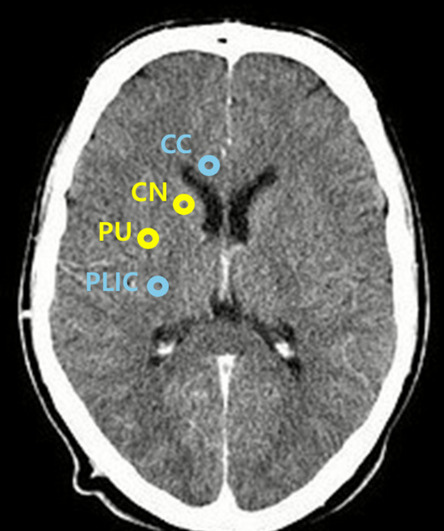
The GWR measurement at the basal ganglia level using brain CT. The calculation methods are as follows: BG-GWR = (CN + PU)/(CC + PLIC); simplified BG-GWR = PU/PLIC. Abbreviations: CT, computed tomography; GWR, gray-to-white matter ratio; BG-GWR, GWR measured at the basal ganglia level; CC, corpus callosum; CN, caudate nucleus; PU, putamen; PLIC, posterior limb of the internal capsule
